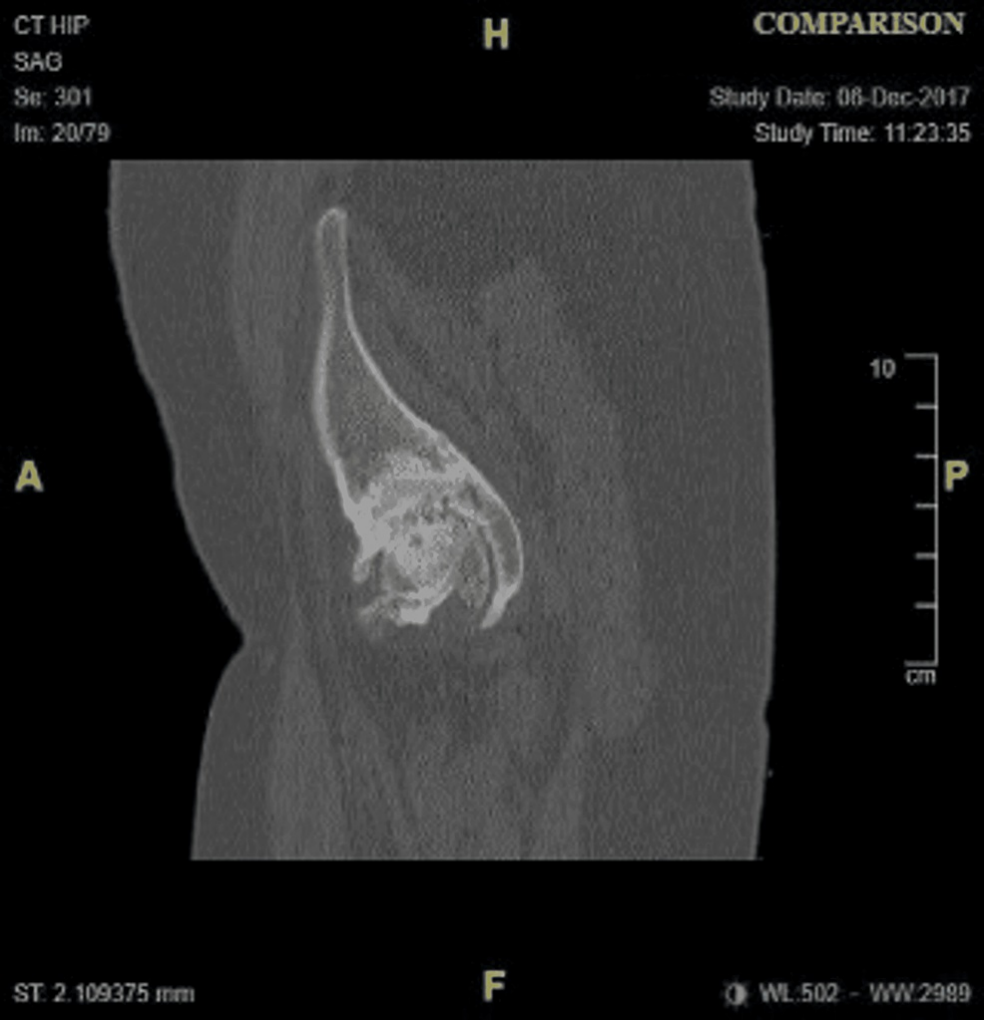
Sagittal CT showing the hip at the time of initial presentation.
